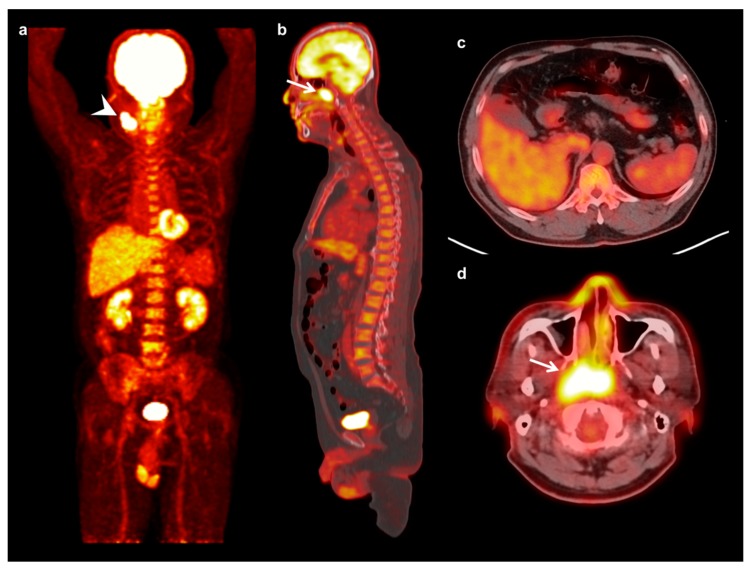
Maximum intensity projection (a) and sagittal (b) FDG PET images and fused transaxial FDG PET/CT images (c,d) of a 57-year-old man with nasopharyngeal cancer. A 5.3 cm-sized mass in the right nasopharynx showed intensely increased FDG uptake (arrow) (maximum SUV 12.61, metabolic tumor volume 44.42 cm^3^, and total lesion glycolysis 237.65 g) with ipsilateral neck lymph node metastases (arrowhead). Serum CRP level, NLR, and PLR of the patients were 6.38 mg/dL, 5.10, and 243.01, respectively. On FDG PET/CT, markedly increased FDG uptake was shown in the bone marrow (BM SUV of 2.37 and BLR of 1.03). The patient was clinically diagnosed with T3N2, stage III, and underwent concurrent chemoradiotherapy. The cancer lesion progressed at 9.1 months after treatment.
